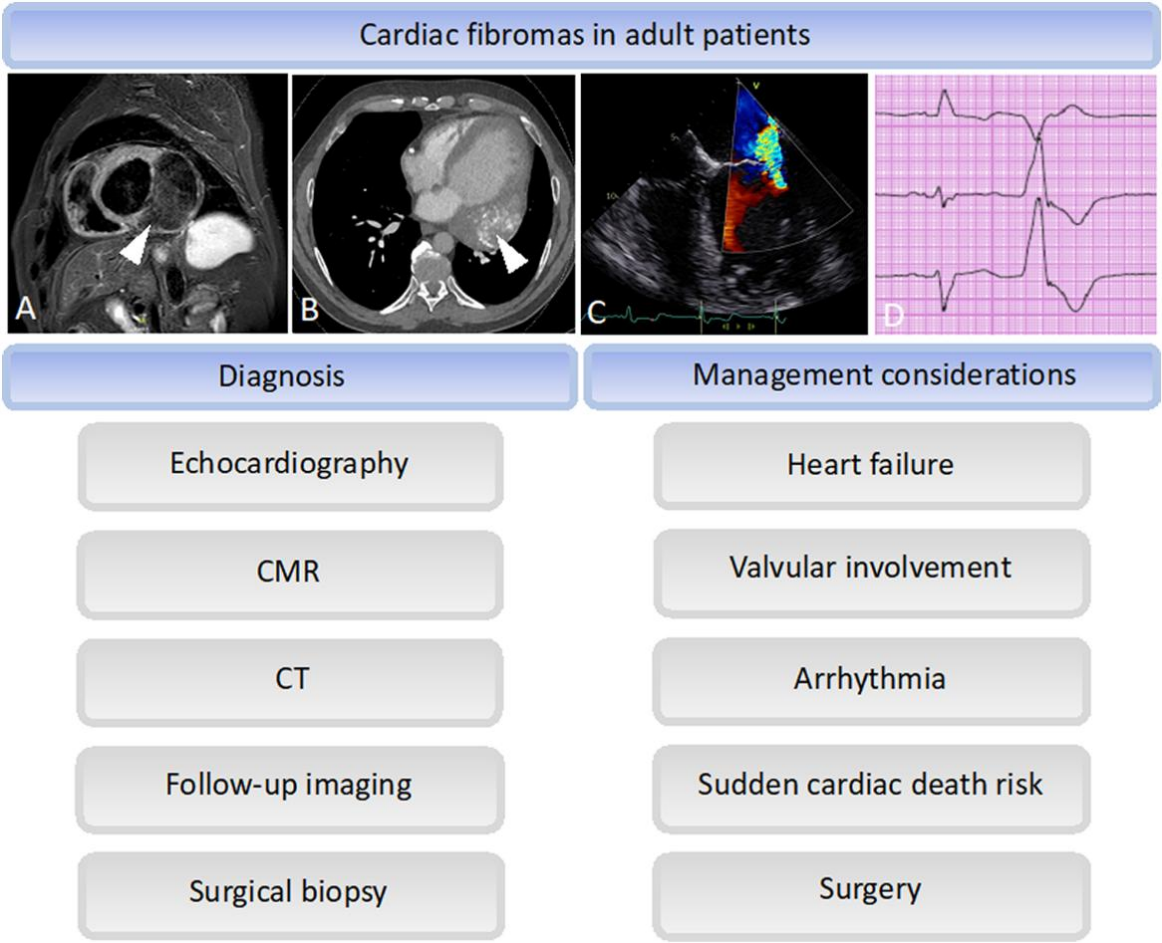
Diagnosis and management considerations of cardiac fibromas in adult patients. Fibromas were diagnosed by CMR in all cases (*A*, example from Case 1). CT supported the diagnosis in Cases 2 and 3 (*B*, example from Case 1). Fibroma led to valvular regurgitation in Case 1 with mitral regurgitation detected by transoesophageal echocardiography (*C*, colour Doppler regurgitation jet over the mitral valve). Premature ventricular contractions were present in Cases 1 and 2 (*D*, example from Case 1). CMR, cardiovascular magnetic resonance; CT, computed tomography.
